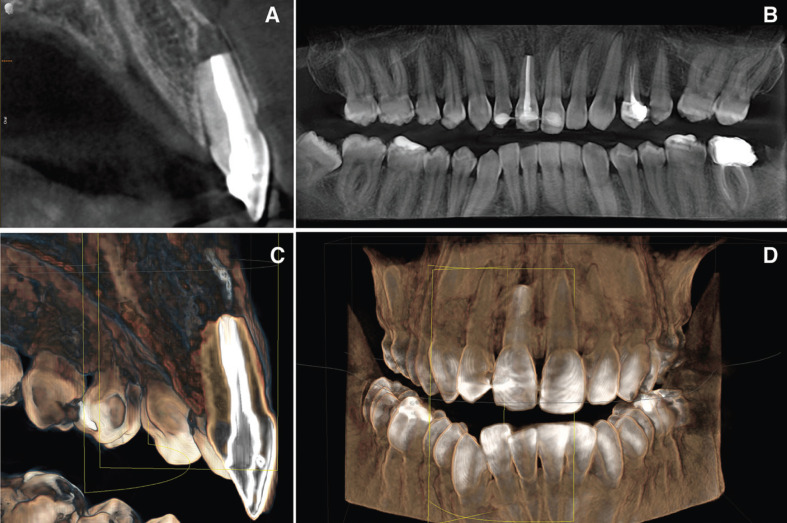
Radiographic study after replantation of the avulsed tooth. A) Axial tomographic cut of the upper right incisor replanted in the socket. B) Orthopantomography after tooth replantation. C) 3D axial reconstruction of the right upper incisor replanted. D) 3D reconstruction of the patient’s bone jaws and teeth after tooth replantation.
